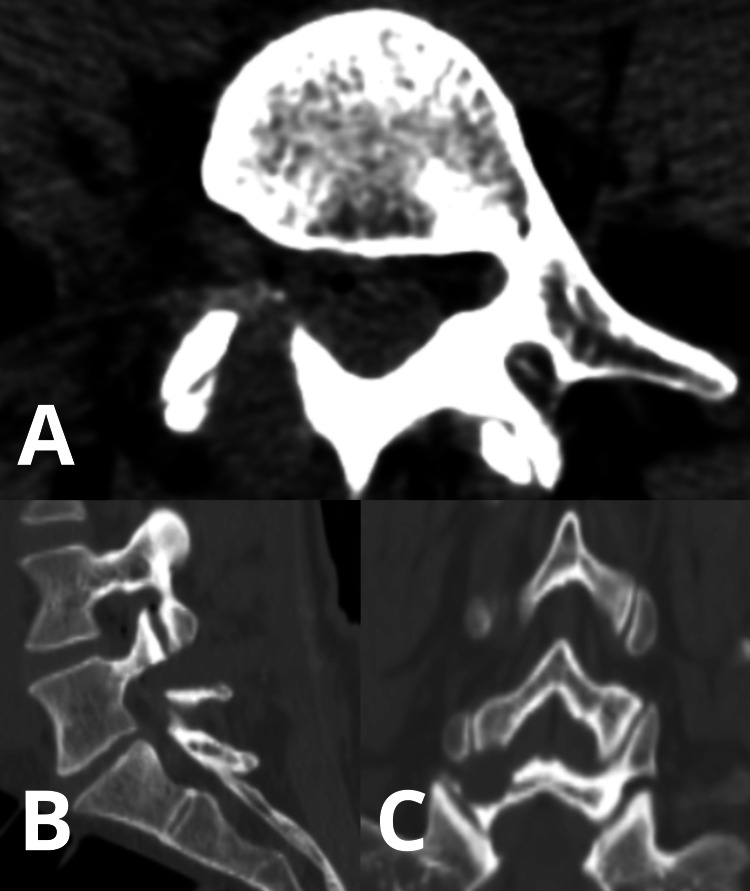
Post-operative lumbar spine CT scan showed no residual compression of the neural structures. The surgical corridor can be explored on the sagittal view. A) Axial view. B) Sagittal view. C) Coronal view.
